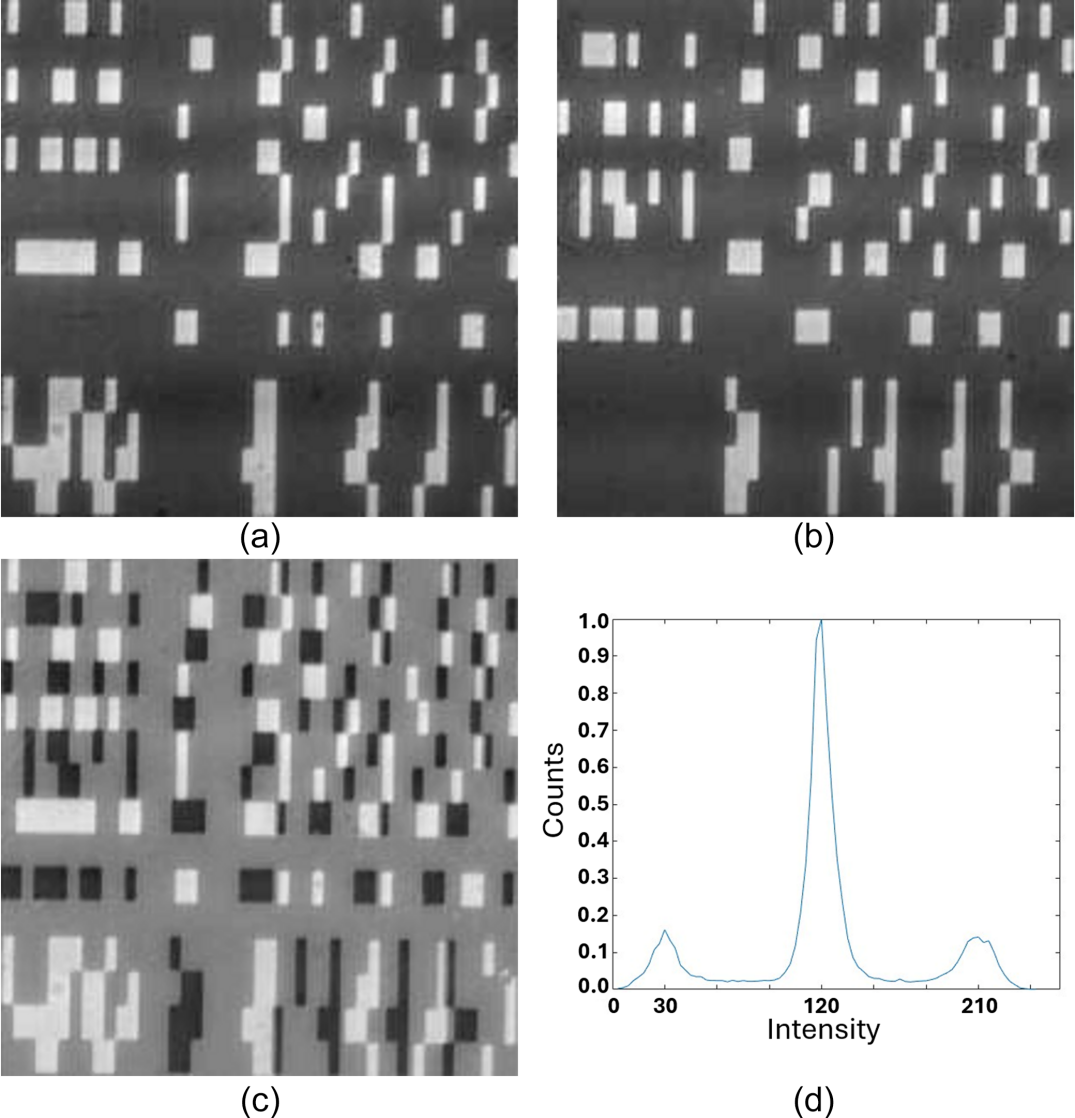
Detector images of the (*a*) positive (*P*) and (*b*) negative (*N*) 15 × 15 pixel Huffman-like mask generated using 3 × 3 binary pixels of pitch 15 µm. (*c*) The signed mask, re-generated as *P* − *N*. (*d*) Histogram of image (*c*). The three peaks show the distribution of the measured X-ray illumination intensities that passed through the −1, 0, +1 sections of the fabricated mask, respectively. The vertical axis in (*d*) is counts; the horizontal axis displays the relative signal intensity.
